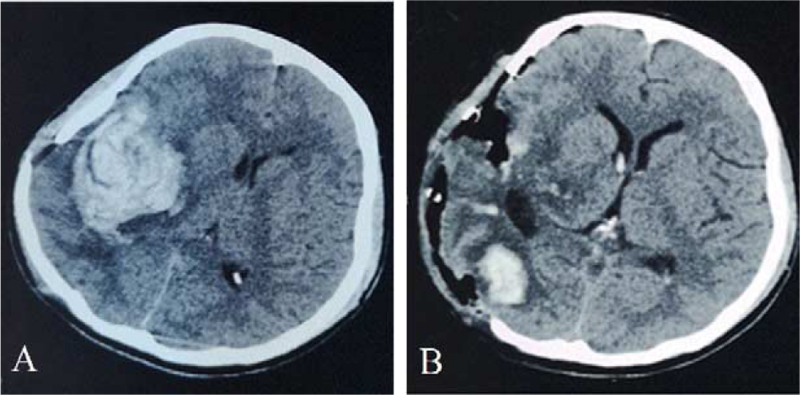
Patient's imaging data during the third ICH. (A) The head CT showed ICH in the right basal ganglia region. (B) Postoperative head CT showed that most of the hematoma was cleared. CT = computer tomography, ICH = intracerebral hemorrhage.
